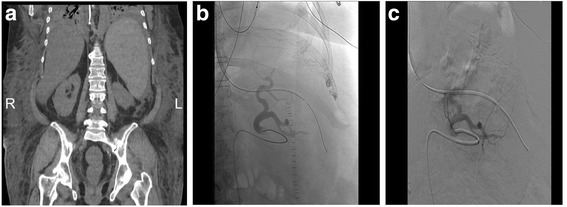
a Coronal views of the patient’s enlarged spleen on CT scan. Arteriogram of the upper pole branch of the splenic artery, performed with CO_2_ gas followed by Visipaque contrast, pre- b and post-embolization c Gelfoam mixed with gentamicin was used to achieve complete stasis
